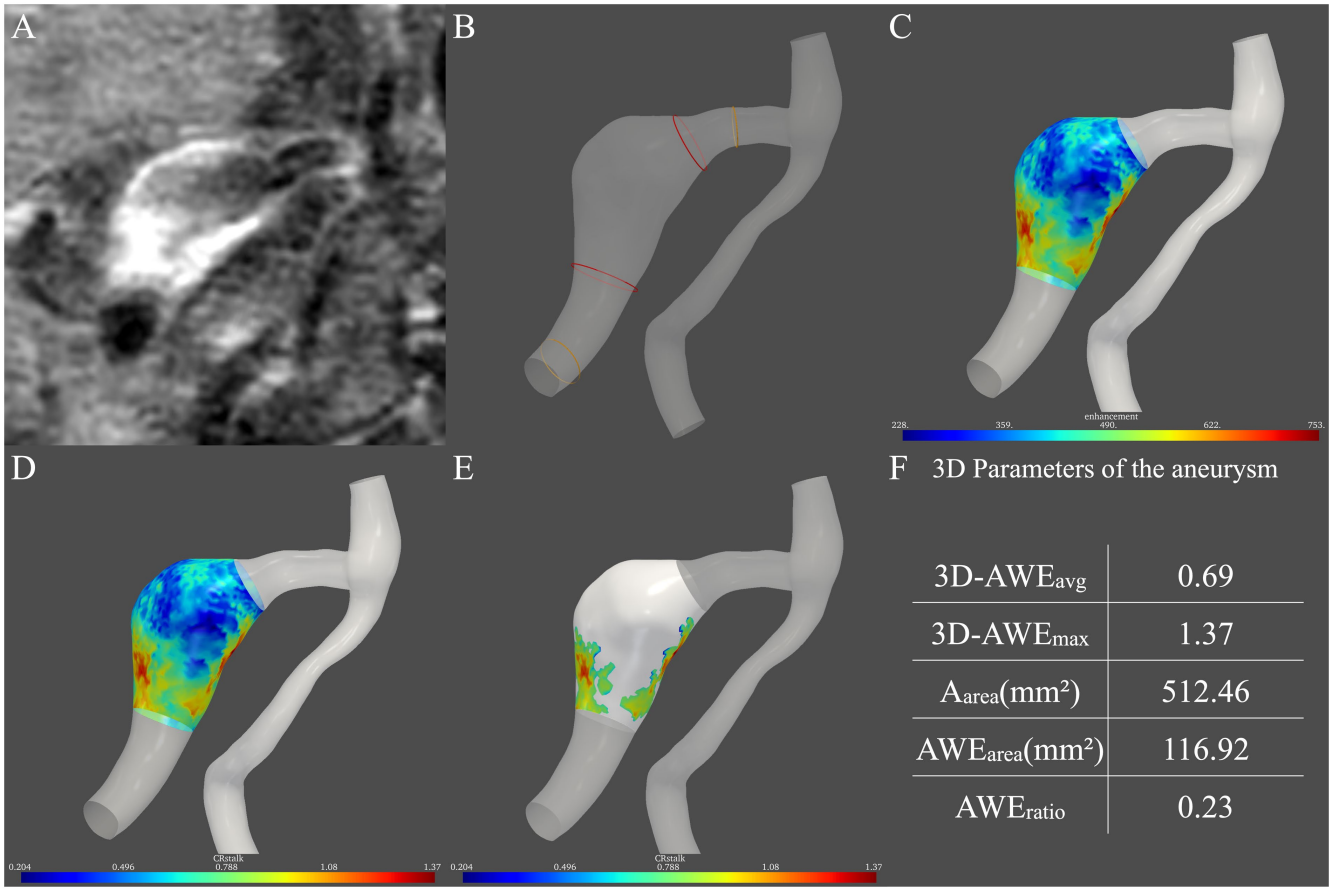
Procedure for measurement of three-dimensional aneurysmal wall enhancement (AWE) on high-resolution magnetic resonance imaging. (A) AWE in the two-dimensional plane was first observed. (B) Then, the two boundaries of the aneurysm (red ring) were defined as 1.5 times the normal vessel (yellow ring) diameter. (C) After the signal intensity (SI) of the post-contrast images was projected onto the model, the SI of the entire aneurysm was obtained. (D) Then, CR_stalk_ was calculated using the SI of the entire aneurysm. (E) Areas with high AWE (>0.9 × SI_stalk_) were then observed. (F) Finally, five indicators in three dimensions were obtained: 3D-AWE_avg_, 3D-AWE_max_, A_area_, AWE_area_, and AWE_ratio_.
